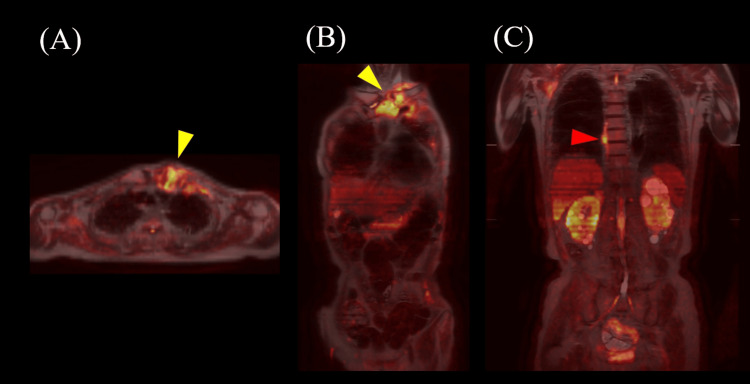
Results of magnetic resonance imaging (MRI) using diffusion-weighted whole-body imaging with background body signal suppression (DWIBS), obtained five months after stroke onset. (A, B, C) DWIBS showing hyperintensities in the left sternoclavicular joint (yellow arrowheads) and along the right edge of the T8 to T9 vertebral bodies (red arrowhead). (A) Axial section; (B, C) coronal sections.
